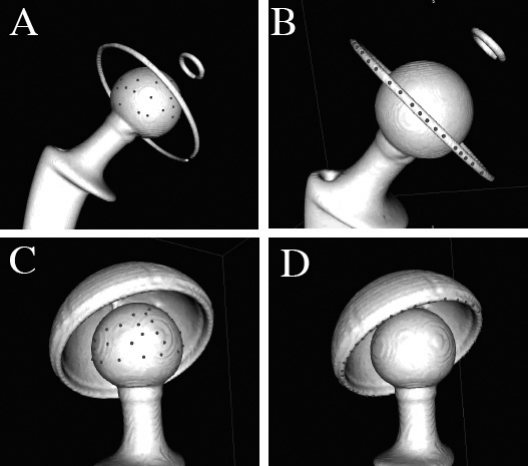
Digitization of points on 3-dimensional image reconstructions of CT- scanned implants. A and B. An all-poly acetabular component assembled with its matching femoral stem and 28-mm diameter head. C and D. A metal-backed PE acetabular component assembled with its matching femoral stem and 32-mm diameter head.
